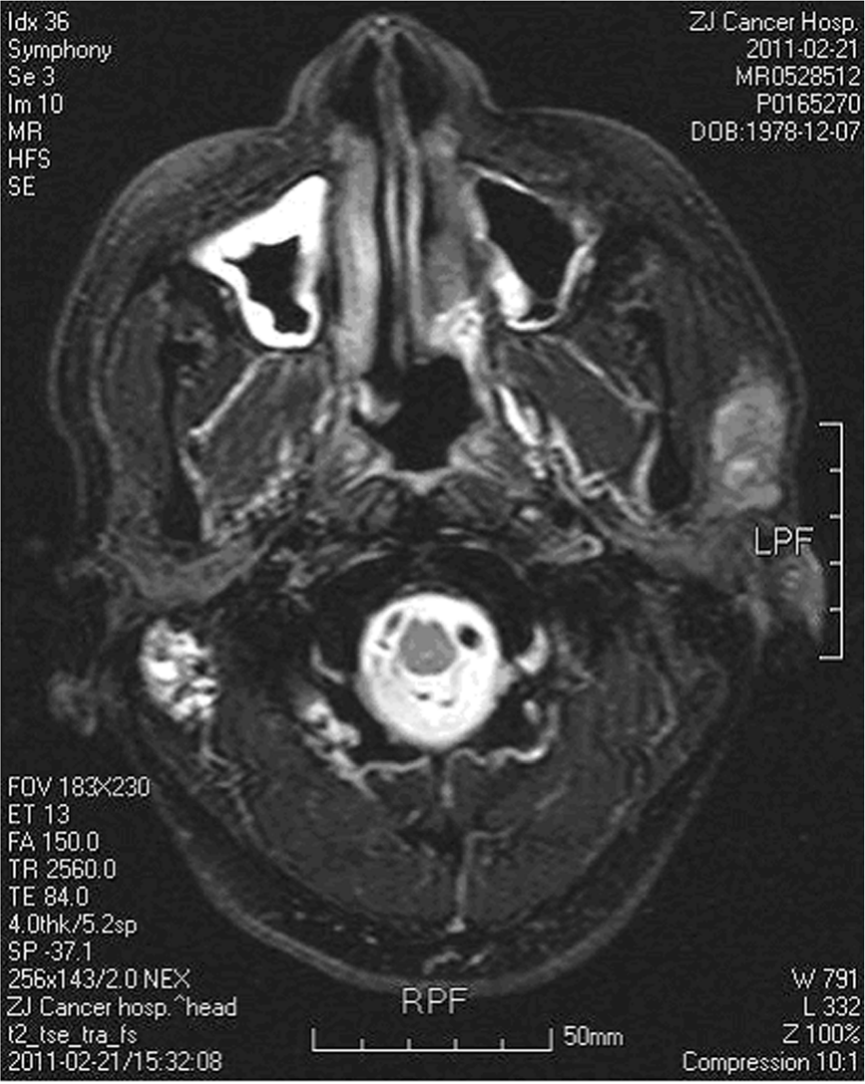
MRI image of delayed parotid metastasis of nasopharyngeal carcinoma after radiotherapy. A nodule about 2.4 × 1.4 cm was observed in the left parotid gland.
